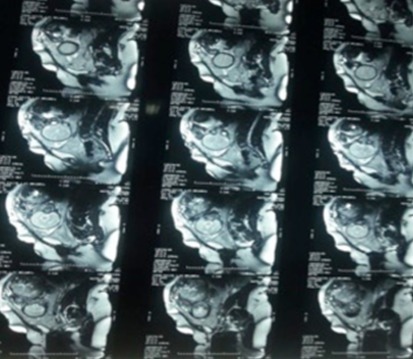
MRI images suggestive of placenta percreta
